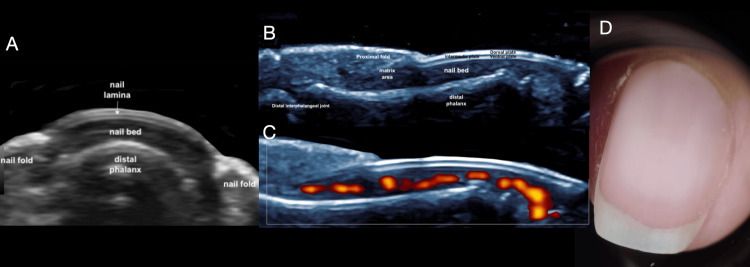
Normal nail anatomy on ultrasound and dermoscopy. (A) Grayscale axial image showing the normal ultrasonographic anatomy of the nail. (B) Longitudinal grayscale image depicting the normal anatomy of the nail. (C) Longitudinal power Doppler image demonstrating normal vascularization of the nail. (D) Dermoscopy image showing the appearance of the normal nail lamina.
